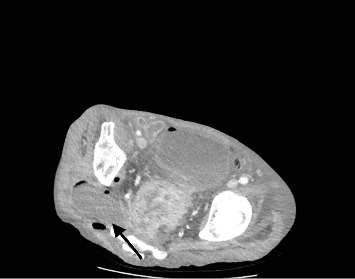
CT of the abdomen showing multiple gas-containing collections in the right gluteal region with the largest one extending into the greater sciatic notch measuring 8 × 4.6 cm.
